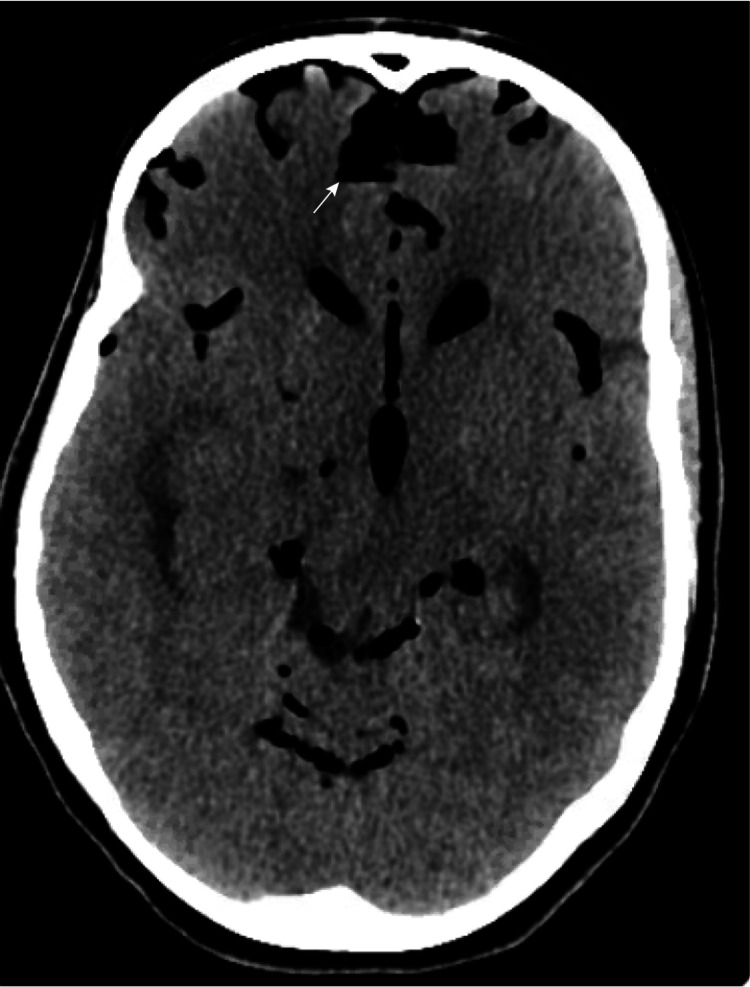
CT brain indicating extensive pneumocephalus five days after initial tumor resection
